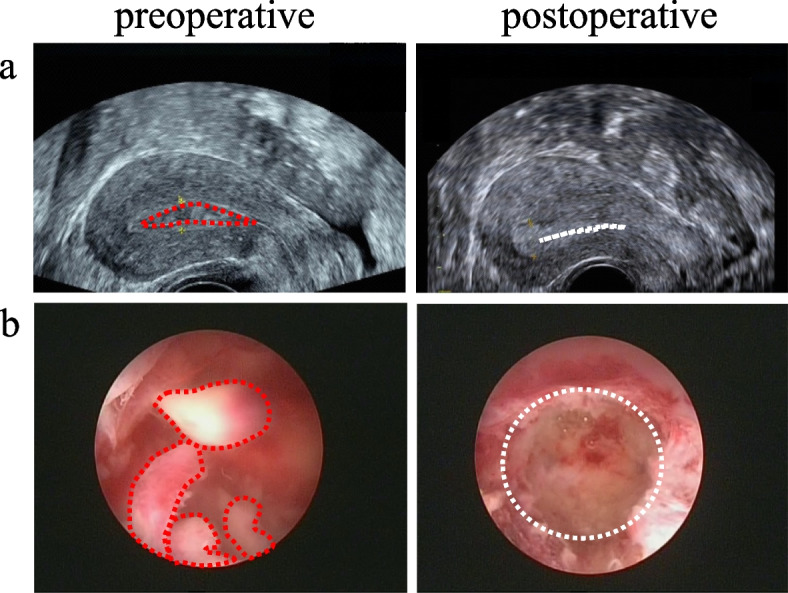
Ultrasound images of the uterus (a) and hysteroscopic view of the uterine cavity (b) before and after hysteroscopic polypectomy. * The red dotted circle represents the outline of the uterine cavity and endometrial polyps before polypectomy. The white dashed circle represents the outline of the uterine cavity after hysteroscopic polypectomy
